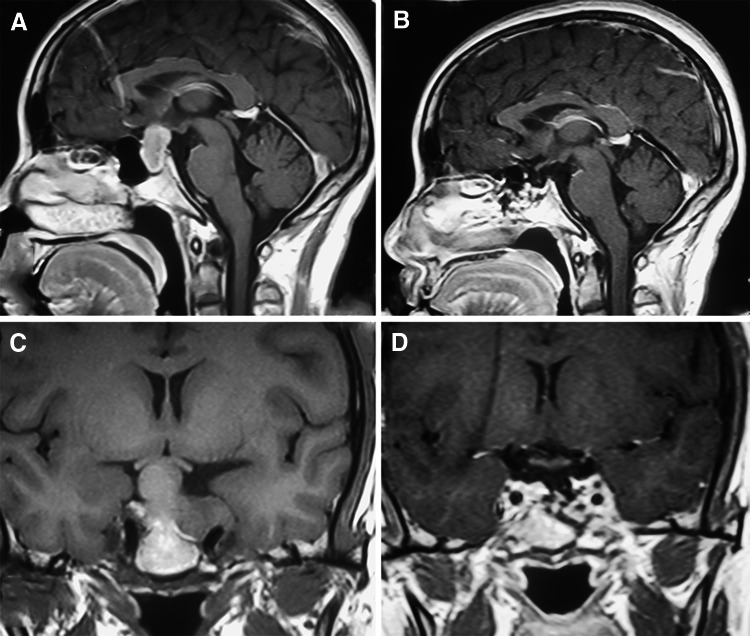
MRI images of one nonfunctioning adenoma invading cavernous sinus. A, C Before surgery, B, D gross total resection after surgery
